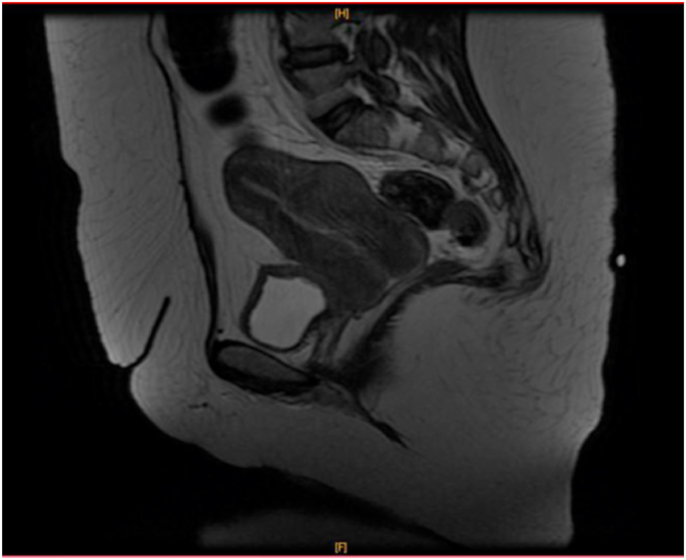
45-year-old patient, metrorrhagia with enlarged uterus on pelvic MRI - sagittal section (T2 sequence): Swollen appearance at cervical-corporeo-isthmic level with deformation of the contours and cavity line, measuring 7 × 5 cm with no pelvic lymphadenopathy. The injection of gadolinium had determined a frank and homogeneous enhancement of the uterine background and a more discreet enhancement in the thickening of the rest of the uterus. With no infiltration of the vagina, bladder, parameters, and dilatation of the two ureters more marked on the right.
